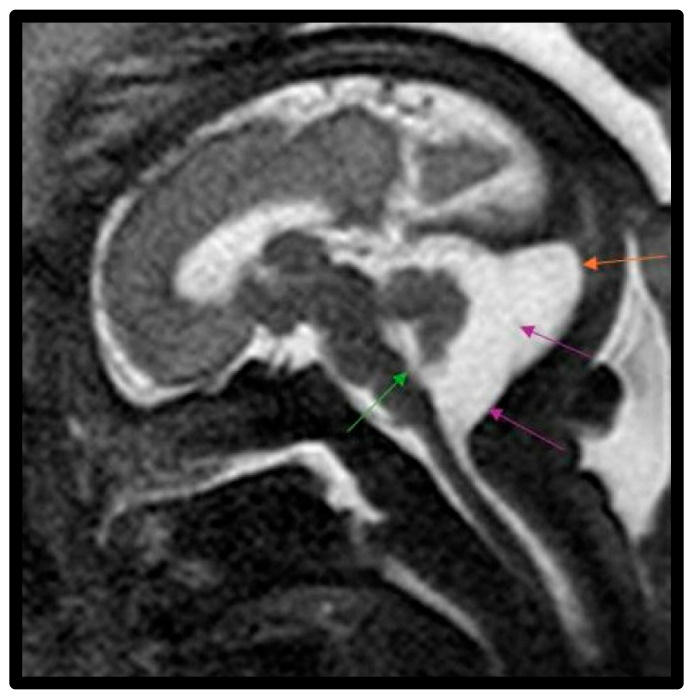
Fetal MRI image of a fetus at 33 gestational weeks with a Blake’s pouch cyst. The T2-weighted sequence in the sagittal plane depicts a slight widening of the midline outlet of the 4th ventricle (green arrow). There is a membrane within the midportion of the CSF space of the cisterna magna with slight bowing of this membrane posteriorly, which is consistent with a Blake’s pouch cyst (purple arrows) involving posterior fossa within this enlarged cisterna magna (orange arrow). The cyst freely communicated with the fourth ventricle with upward displacement of the vermis and elevation of the tentorium.
